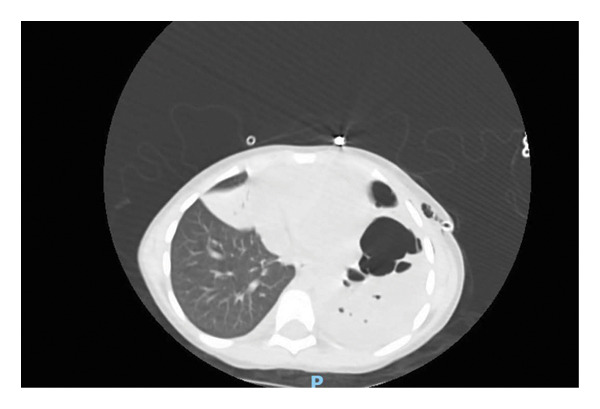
Axial contrast‐enhanced CT scan on admission showing left lower lobe consolidation with areas of hypoattenuation and internal air locules, consistent with necrotizing pneumonia. A large left pleural effusion is also present.
